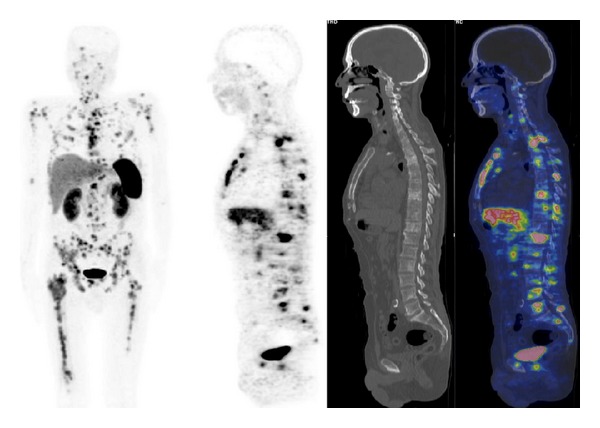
^68^Ga-DOTATOC PET/CT images (MIP, sagittal) in a patient with metastatic small cell lung carcinoma.
